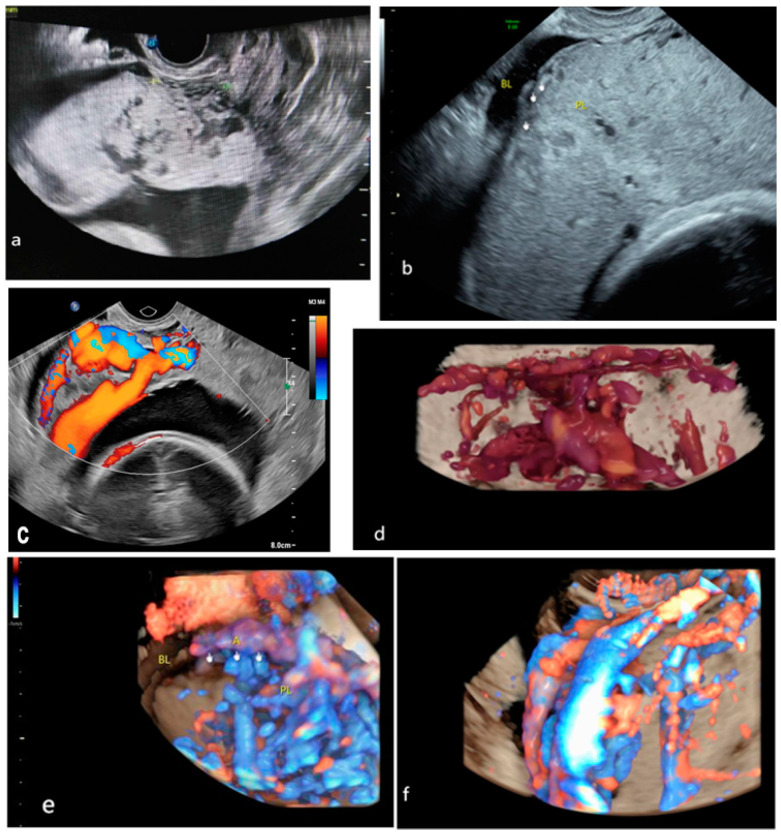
Common traditional ultrasound signs: (a) placental lacunae; (b) myometrial thinning; (c,d) bladder wall interruption; (e,f) bridging vessels. BL, bladder. A, artery. PL, placenta.
